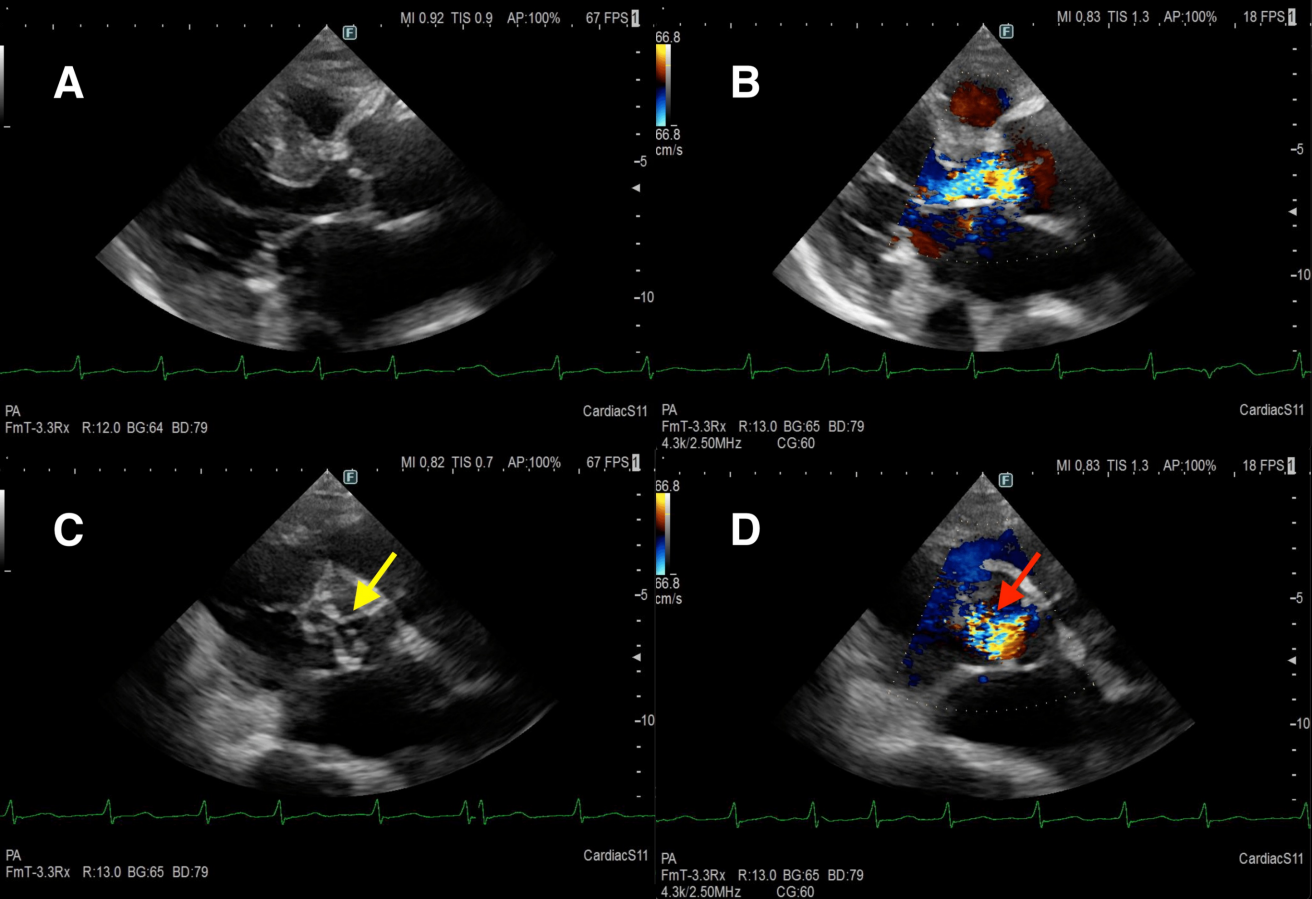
Transthoracic echocardiography Parasternal long‑axis (A, B) and short‑axis (C, D) transthoracic echocardiographic views were obtained on admission day. (A) Two-dimensional parasternal long-axis view shows no obvious valvular vegetations. (B) Color Doppler parasternal long-axis view demonstrates severe aortic regurgitation with an eccentric regurgitant jet. (C) Two-dimensional parasternal short-axis view shows incomplete coaptation of the left coronary cusp (yellow arrow). (D) Color Doppler parasternal short-axis view demonstrates an eccentric regurgitant jet originating near the left coronary cusp commissure (red arrow).
